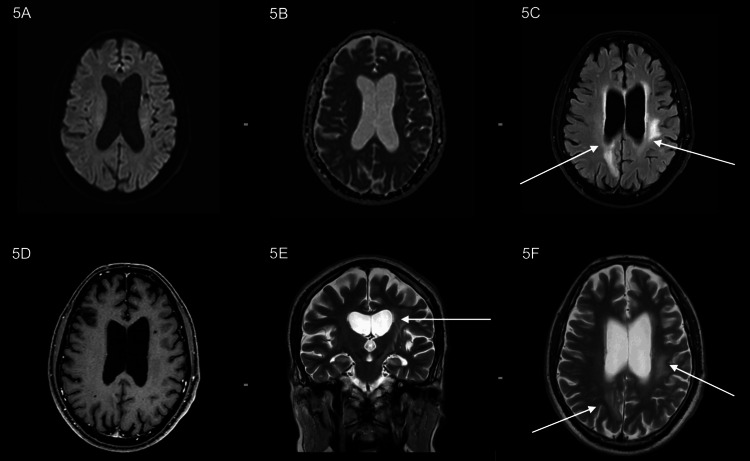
(A, B) DWI and ADC weighted images showing no significant diffusion restriction. (C) Axial FLAIR images showing hyperintensity in parietal parasagittal cortex and left periventricular white matter. (D) Axial post-contrast T1-weighted images showing no evidence of contrast enhancement. (E, F) Coronal and Axial T2 images showing T2 heterogeneous signal intensity with T2 hyperintense oedema predominantly along the left periventricular white matter, left gangliocapsular region, left cerebral peduncles and brainstem. Compared to the prior study, as seen in Figure 3: Significant interval decrease in the size and extent of lesions with resolution of diffusion restriction. DWI: diffusion-weighted imaging; ADC: apparent diffusion coefficient; FLAIR: fluid-attenuation inversion recovery.
